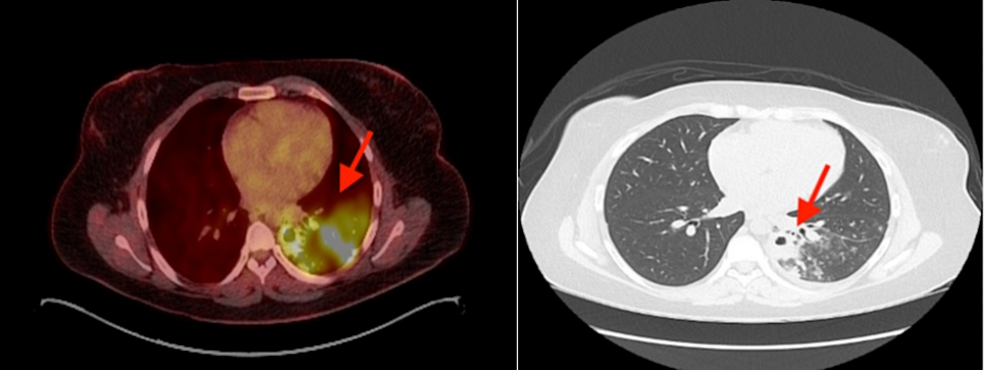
Chest positron emission tomography–computed tomography (PET-CT) showing significant pulmonary infiltrate with cavitary lesions.
